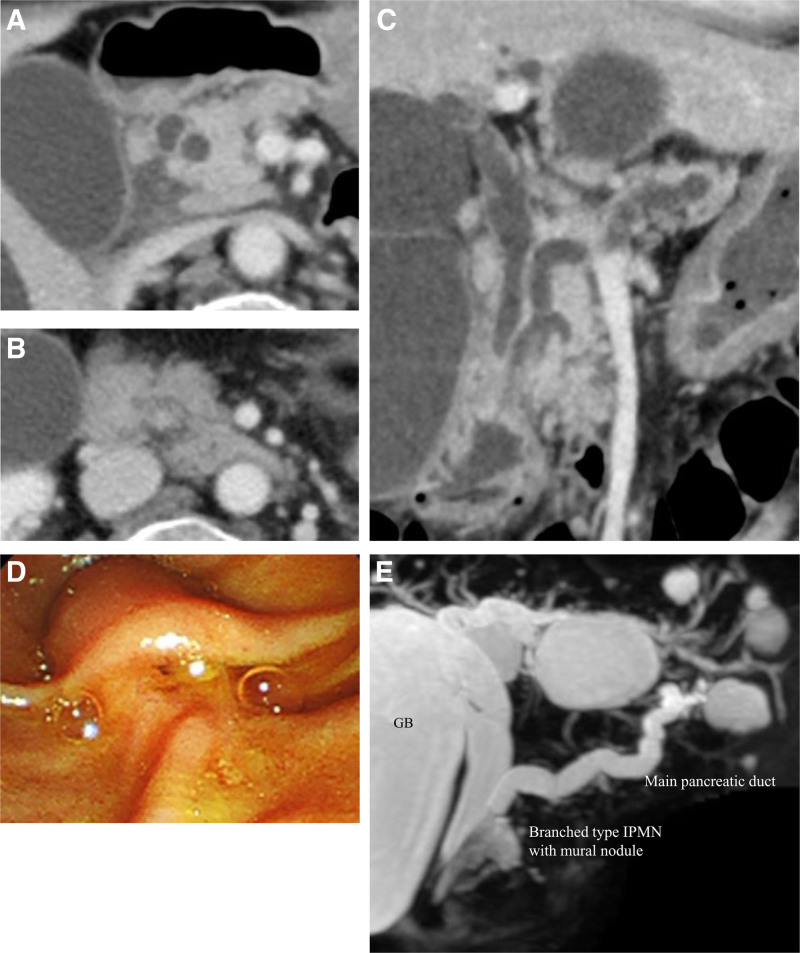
Images at initial diagnosis of intraductal papillary mucinous neoplasm (IPMN). (A) Computed tomography scan image. Axial image of the dilated common bile duct. (B) Axial image of the branch duct-type IPMN and the enhanced mural nodule of the pancreatic head. (C) Coronal image of the dilated main pancreatic duct and common bile duct. The common bile duct wall was thickened. (D) The endoscopic image of the papilla of Vater. (E). Followed up magnetic resonance cholangiopancreatography image revealed a dilated main pancreatic duct with a branch duct-type IPMN and a mural nodule. 12 months after initial diagnosis.
